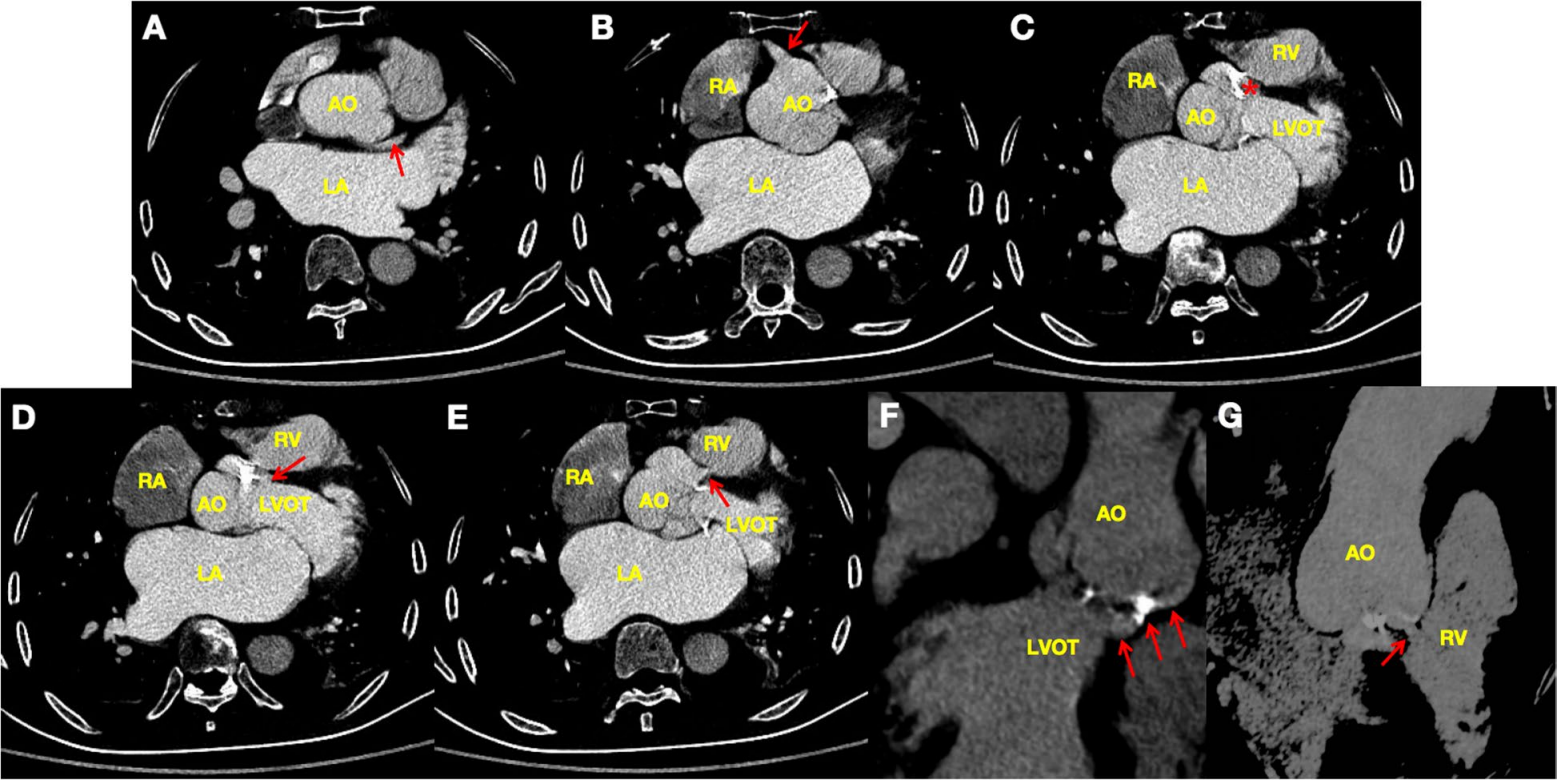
CTA images. A Origin of the LCA (arrow) from the left coronary sinus. B Origin of the RCA (arrow) from the right coronary sinus. C An AVT (asterisk) is anteriorly and laterally located to the right coronary sinus. D The LV opening (arrow) of the AVT was in the left ventricular outflow tract. E The RV opening (arrow) of the AVT was in the right ventricular outflow tract. F The AVT (arrow) was shown by 3D reconstruction. G The RV opening (arrow) was shown by 3D reconstruction
